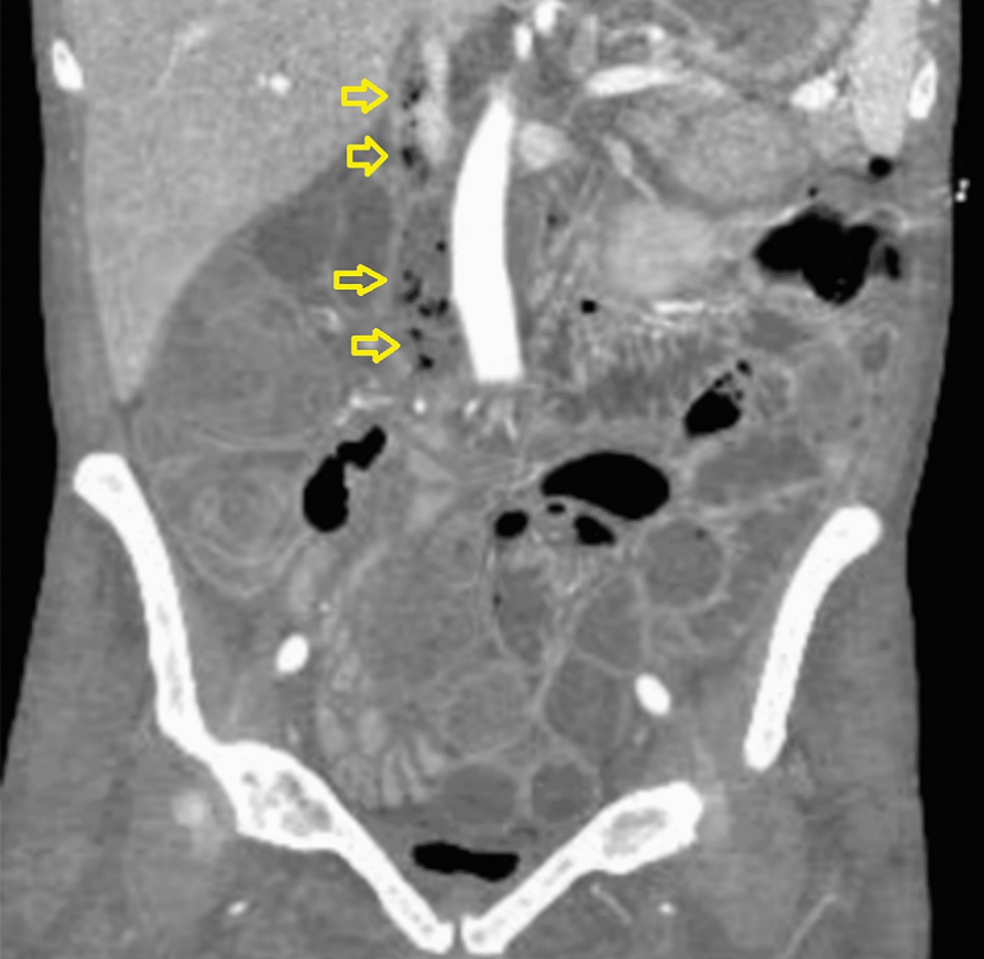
Computed tomography scan of the abdomen and pelvis view showing hypodensities and locules of air (arrows) within the intrahepatic and infra hepatic inferior vena cava.
